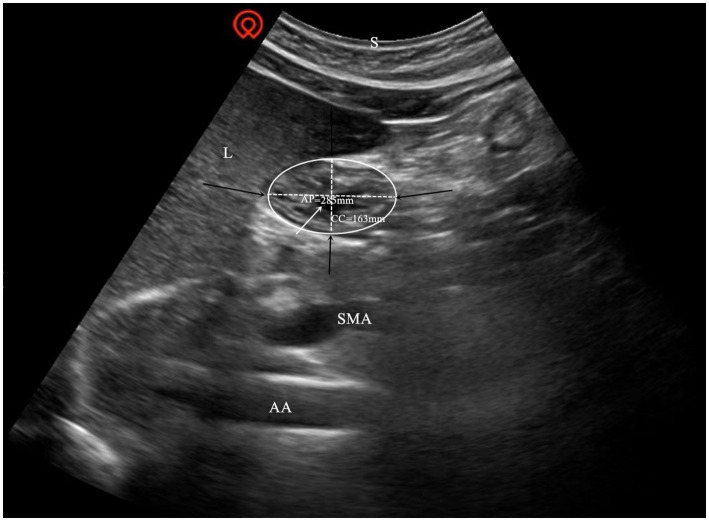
Ultrasound scan of the gastric antrum in the right decubitus position in a subject who was classified as Perlas 1. AP, antero posterior diameter; CC, craniocaudal diameter; S, skin; L, liver; SMA, superior mesenteric artery; AA, abdominal aorta. AP = 285 mm, CC = 163 mm.
